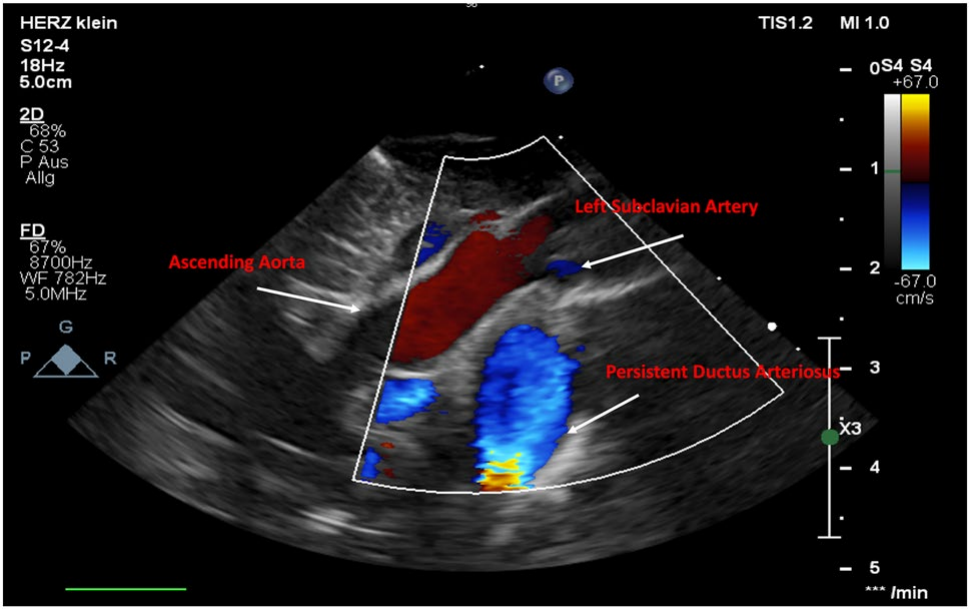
Echocardiography (suprasternal view) showing aortic arch interruption type A (IAA). Systemic circulation was secured by the Persistent ductus arteriosus (PDA)
